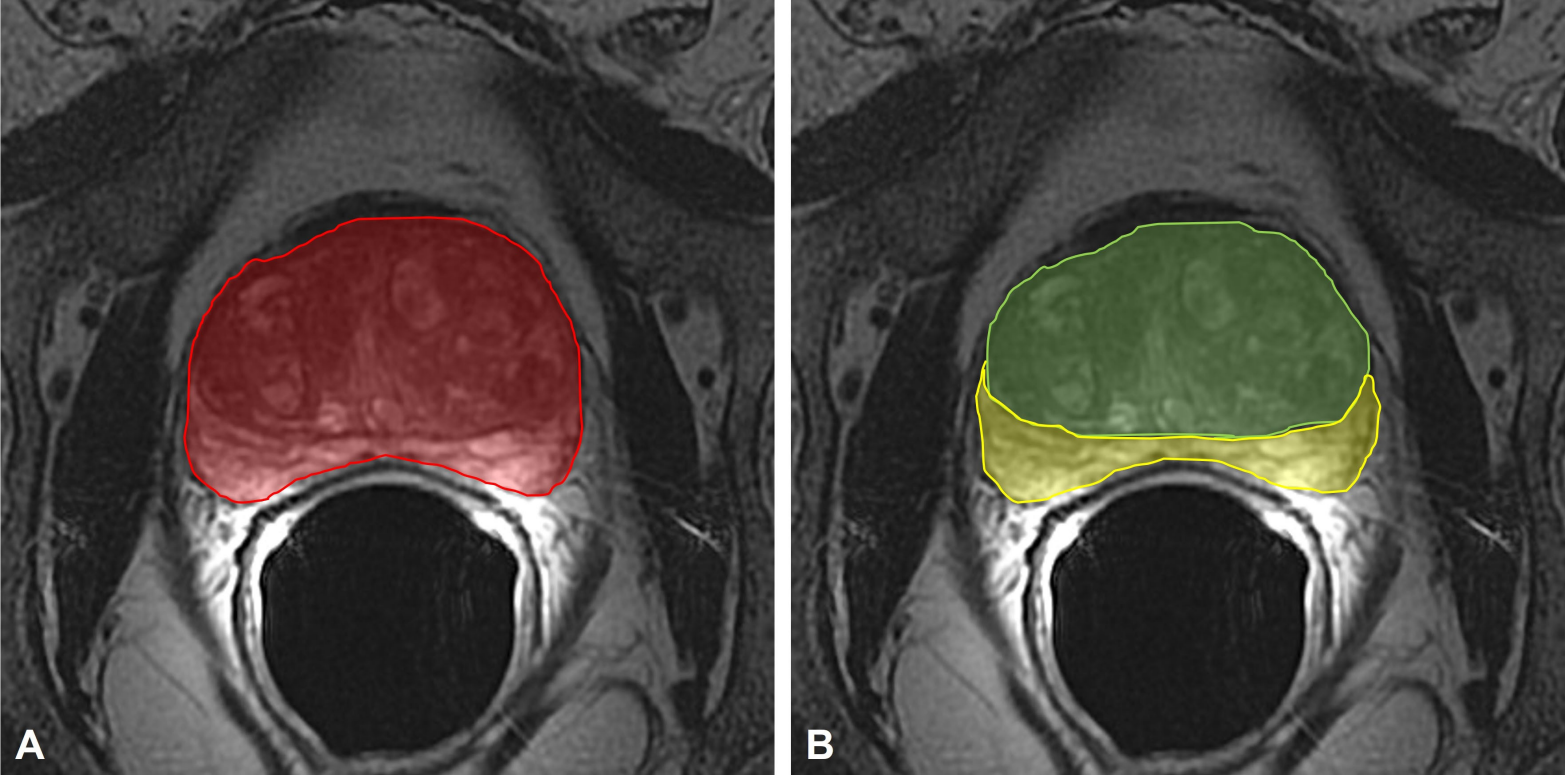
(A) measurement of the TransPA (red area) on the axial FR-FSE T2-weighted maximum sectional area of the prostate; (B) measurement of the TransCGA (green area) and TransPZA (yellow area) on the axial FR-FSE T2-weighted maximum sectional area of the prostate. TransPA, transverse prostate maximum sectional area; TransCGA, transverse central gland sectional area; TransPZA, transverse peripheral zone sectional area; FR-FSE, Fast Relaxation Fast Spin Echo.
